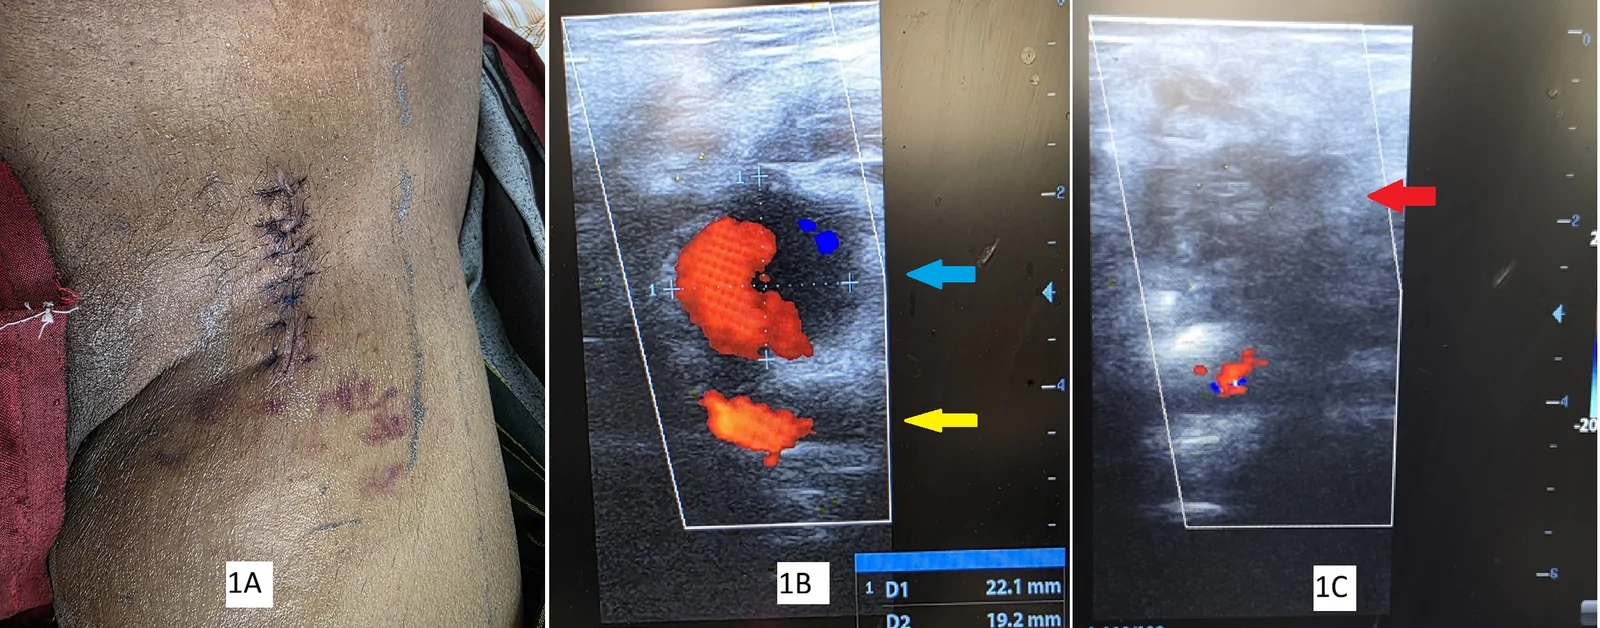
(A) Groin incision without features of infection; (B) False aneurysm on duplex ultrasound (Blue arrow: aneurysm, Yellow arrow: vein graft); (C) Thrombosed false aneurysm after percutaneous fibrin sealant injection (Red arrow).
